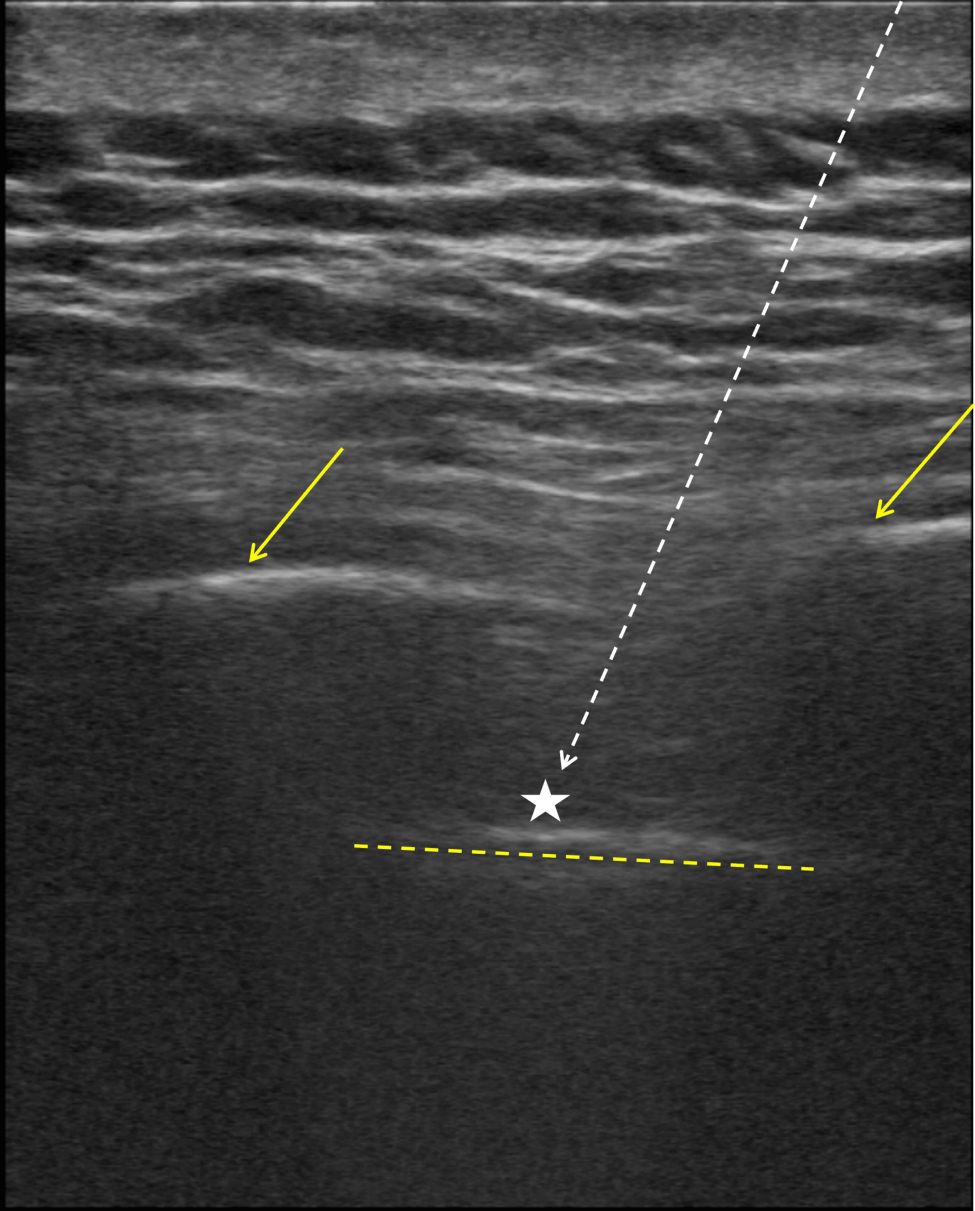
Ultrasound-guided intercostal nerve block. A 25-gauge spinal needle (limited needle visualization; white dashed arrow) is advanced with a caudal to cranial angulation into the subcostal groove (star), positioned just superior to the pleura (yellow dashed line) and between adjacent ribs (solid yellow arrows)
